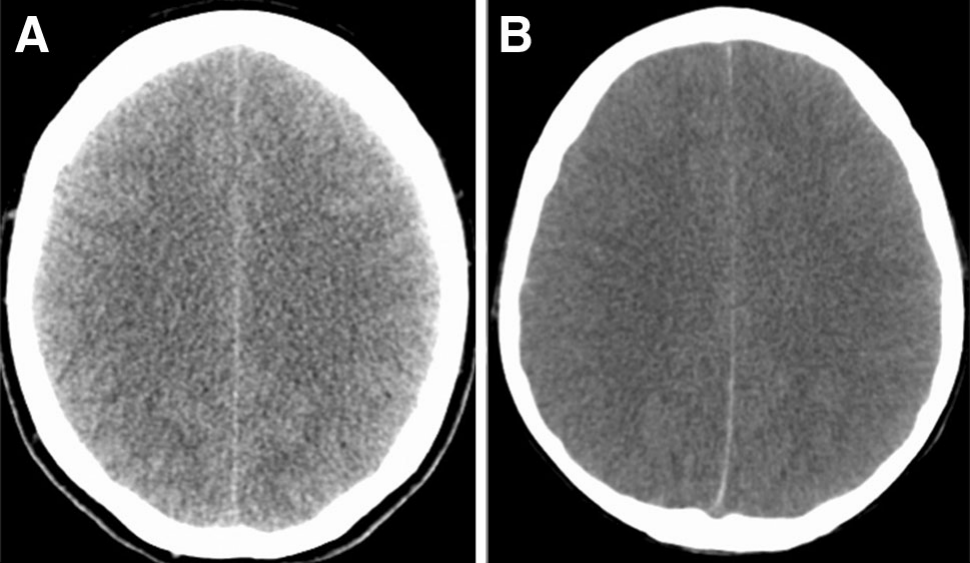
Axial computed tomography (CT) scans on admission demonstrating global cerebral edema in patient one (a) and two (b)
